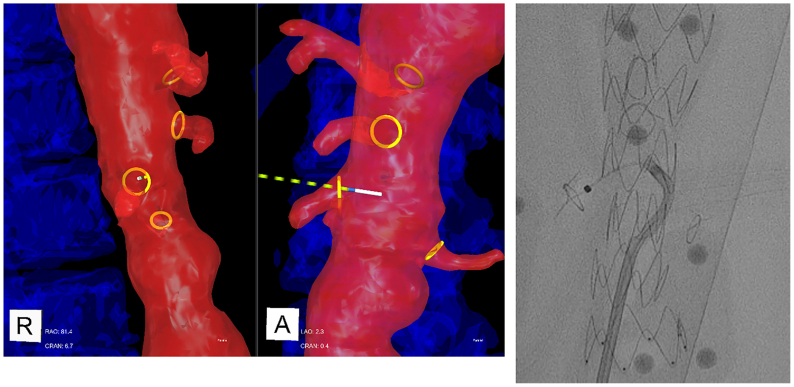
Intraoperative positioning system (IOPS) navigation (*left*) and confirmation angiogram (right) demonstrating position of IOPS guidewire inside right renal artery (RRA) confirming fenestration position. IOPS fiducial markers are visible in the radiograph.
